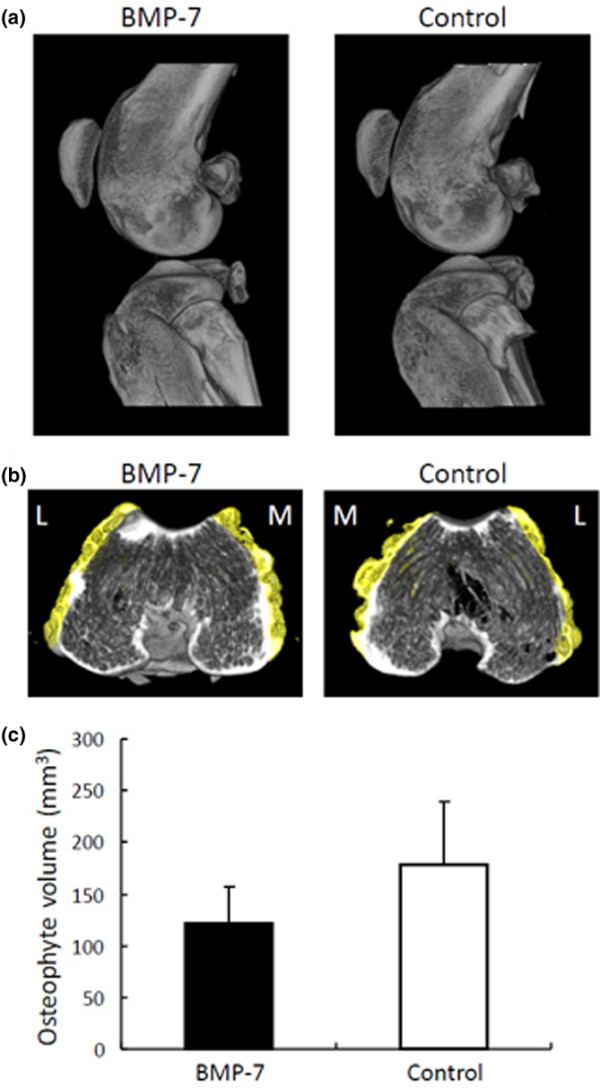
Evaluation of ectopic bone and osteophyte formation. After anterior cruciate ligament transection in both knees, 500 ng BMP-7 in 200 μl PBS was injected weekly into the right knee and the same amount of PBS was injected into the left knee. Knee joints were analyzed using a micro CT scanner at 12 weeks. (a) Representative three-dimensional CT images of BMP-7 treated and control knee. Lateral views show no obvious ectopic bone formation in BMP-7 injected side and control side. (b) Representative reconstructed CT images of distal femoral osteophyte. Osteophytes were colored with yellow by image processing. Laterality is shown as lateral (L) and medial (M). (c) Quantitation of osteophyte volume. The values are displayed as average ± standard deviation (*n* = 5). BMP, bone morphogenetic protein; CT, computed tomography; PBS, phosphate-buffered saline.
